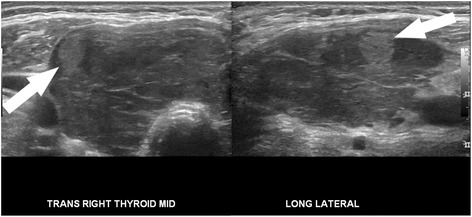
Very low suspicion pattern. Transverse and longitudinal sonographic images of the right lobe of the thyroid show an echogenic nodule (*arrow*) against a background of hypoechoic, enlarged thyroid with curvilinear echogenic bands. These features of the background thyroid are characteristic of Hashimoto’s thyroiditis. The echogenic nodule is a benign “white knight” nodule, representing a regenerative nodule
